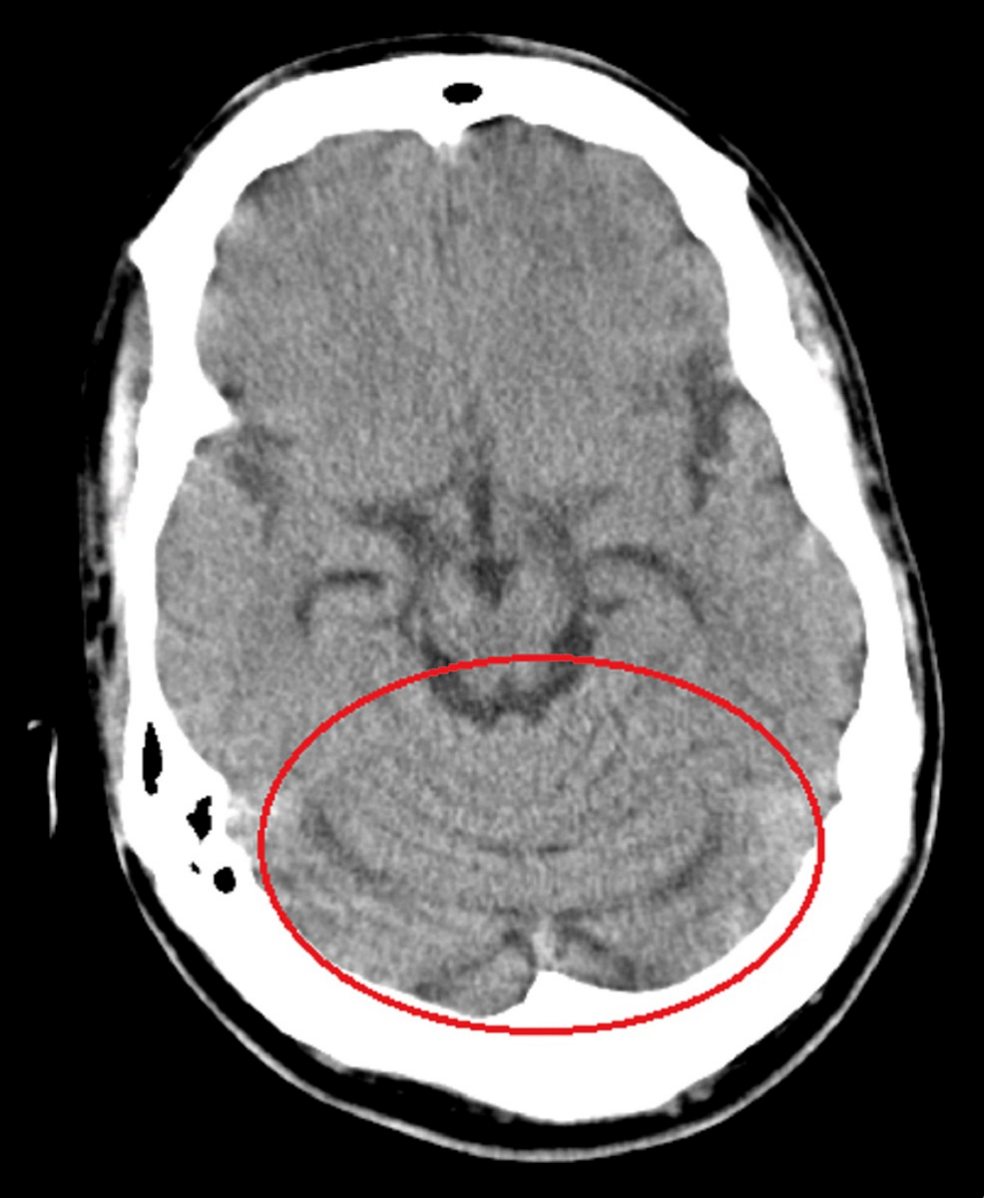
Brain computed tomography revealing cerebellar atrophy (red circle).
